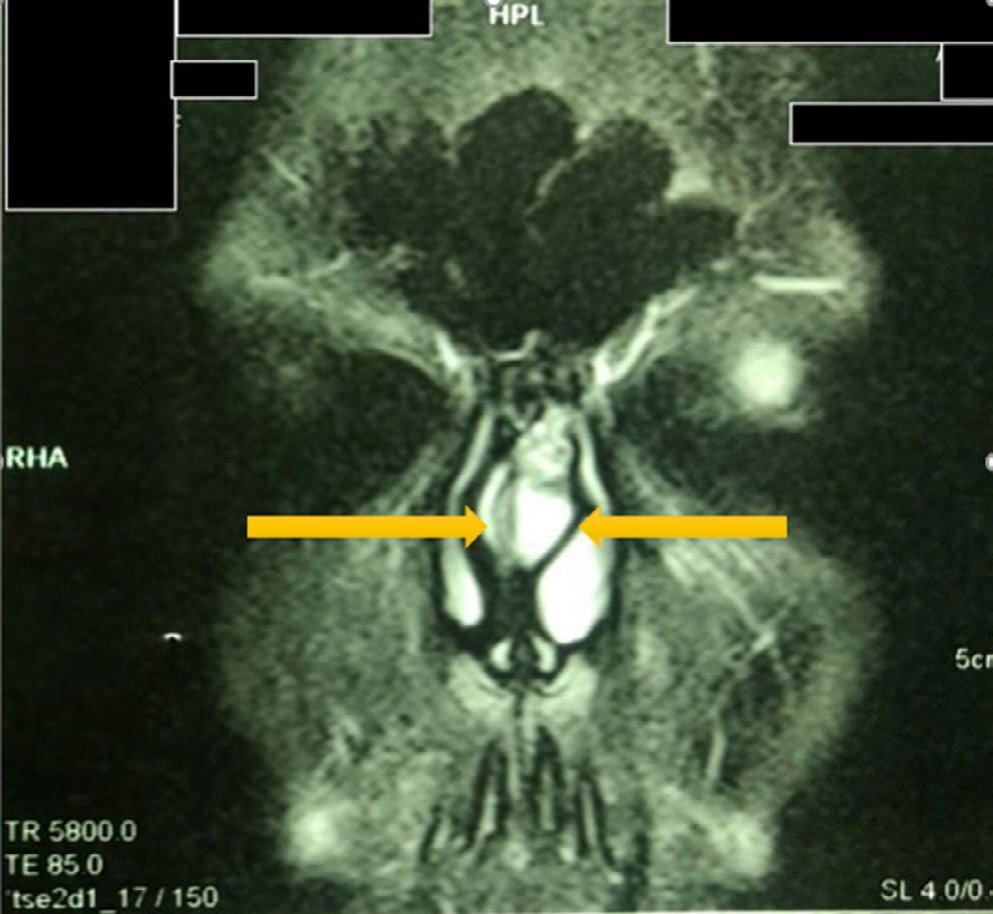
T2‐weighted MRI shows bizarre‐shaped 54*8*94 mm hyper signal mass located in left anterior part of the nasal septum
